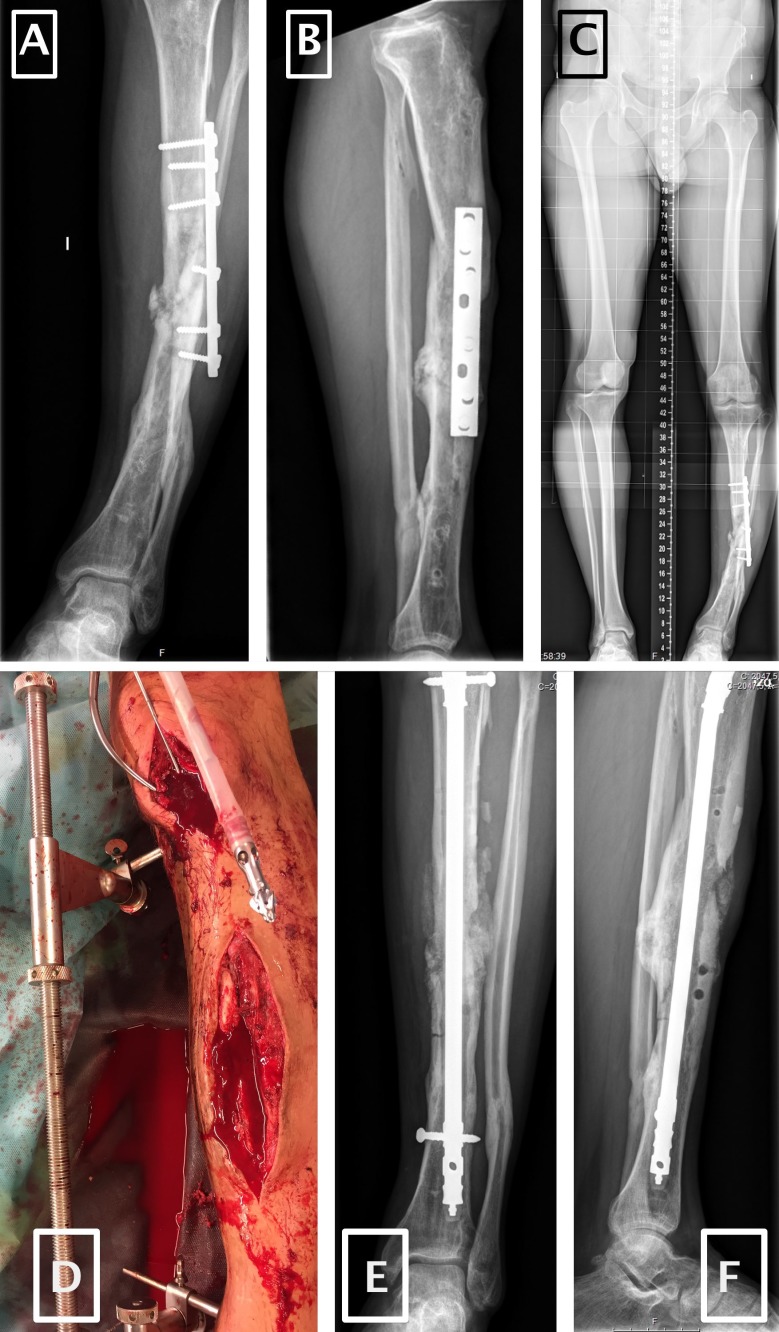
Images that show the reconstructive steps of a tibial non-union. Images A and B: Anteroposterior and lateral X-ray. Image C: Teleradiograph in full-weight. Image D: Intraoperative correction with a distractor and RIA reamer. Images E and F: postoperative anteroposterior and lateral X-ray after non-union treatment.
